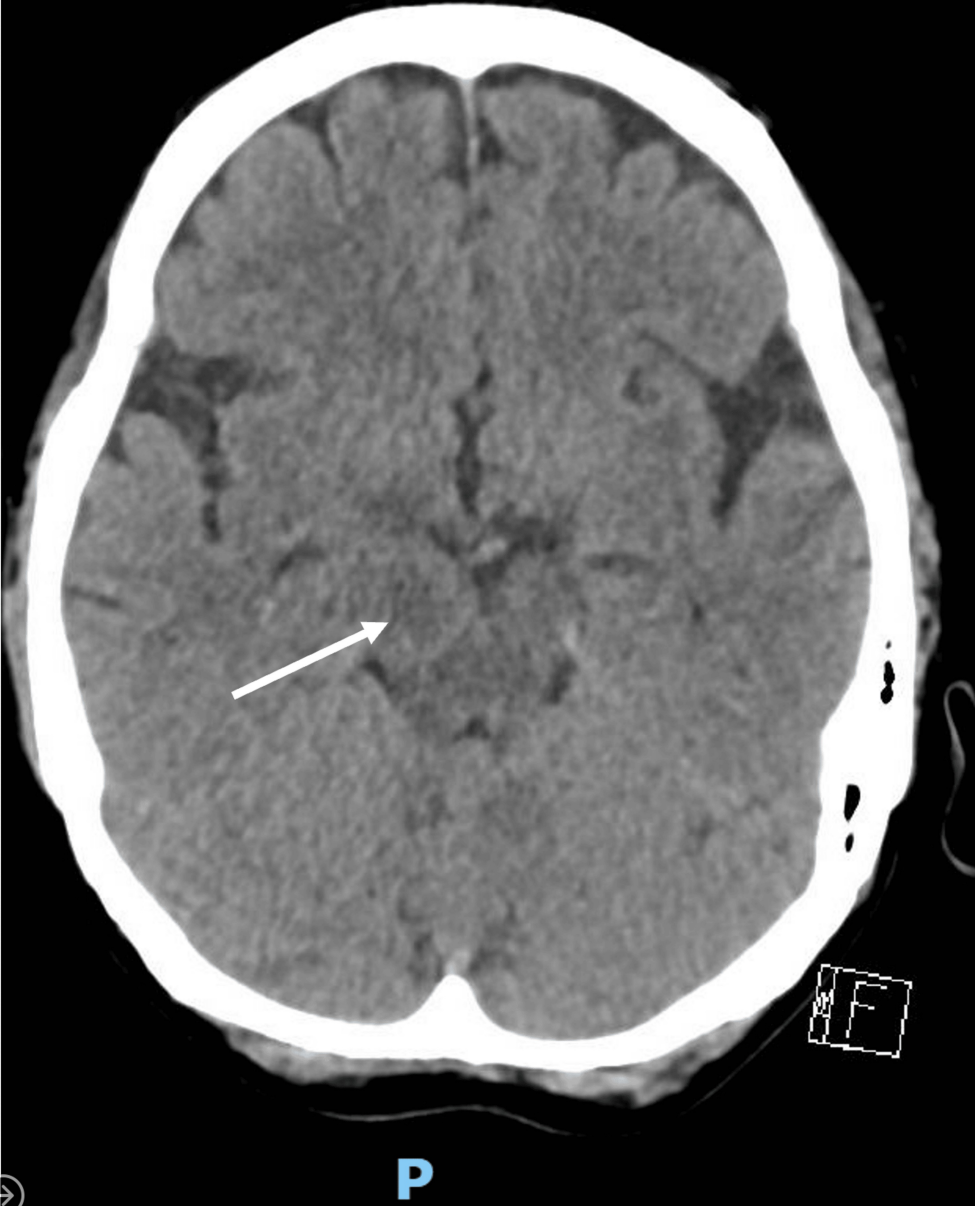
Non-contrast enhanced CT scan of the brain performed on admission demonstrating right cerebral peduncle hypodense lesion (arrow)
